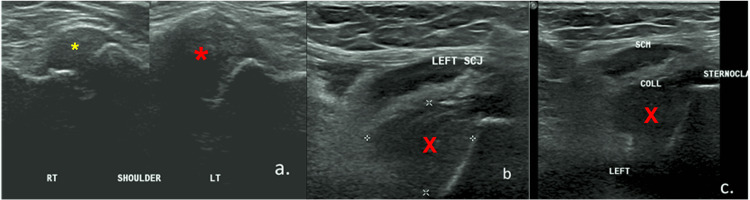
(a) Ultrasound of the sternoclavicular joint showed a distended capsule on the left side (big red asterisk) when compared to the right (small yellow asterisk). (b & c) Ultrasound revealed a collection (red 'X') inferior to the left sternoclavicular joint which extended into the left thoracic region.
